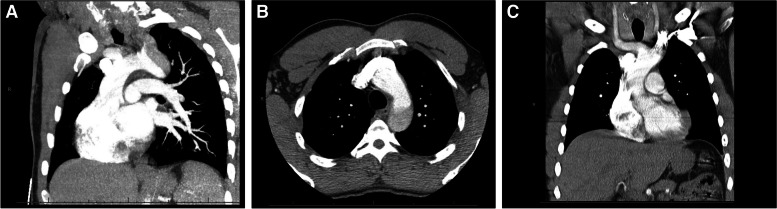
A-C Initial presentation CT angiogram with Zone 3 aortic dissection with retrograde extension into left subclavian artery (1A: Sagittal cross-section; 1B: Axial cross-section; 1C: Coronal cross-section)
